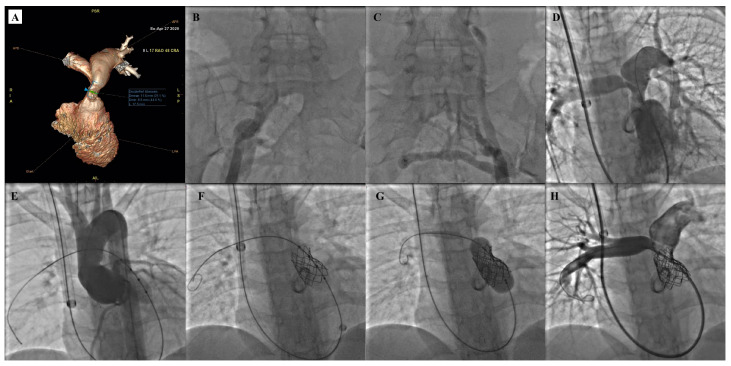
Procedural steps of Melody TPV implantation. Angio-CT scan detection of a significant stenosis of an RV-PA conduit (A). Bilateral thrombosis of femoral veins (B,C) forced them to adopt the right jugular vein as vascular access. Pulmonary artery injection highlights a significant stenosis of the RV-PA conduit (D). RVOT balloon interrogation identifies the lack of coronary artery compression (E). RV-PA conduit pre-stenting (F) is followed by Melody TPV implantation (G). Pulmonary artery injection highlights a well-deployed continent Melody TPV (H).
